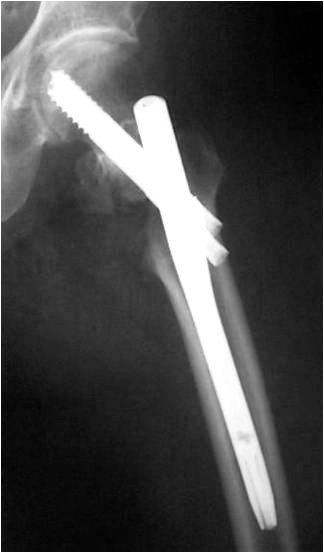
At final x-rays, the 2 proximal screws were inserted slightly convergent and retroverted. The femoral head reduced in slight valgus and gap at the medial site of the fracture is noticed at final x-rays.
